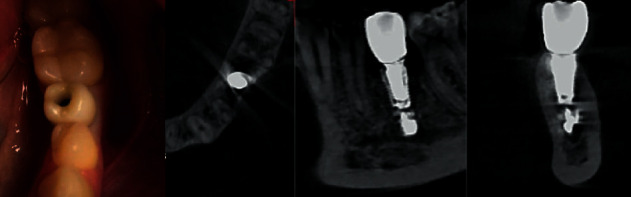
Fixing a permanent structure. CBCT. After the final restoration, the implant is also located in compliance with the safety zone.
